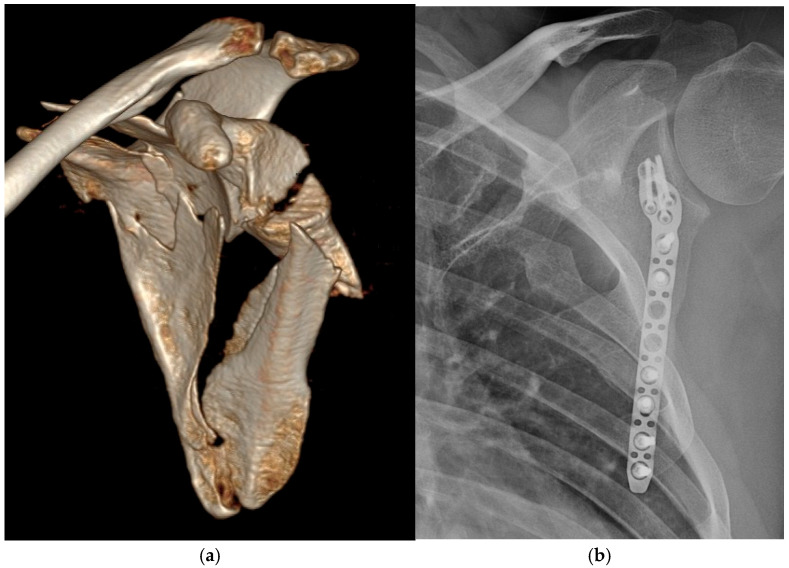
(a) Preoperative three-dimensional computed tomography demonstrates a fracture extending from the glenoid fossa to the scapular body. (b) Postoperative radiograph shows stable fixation with a lateral border plate and screw fixation of the glenoid fragment.
